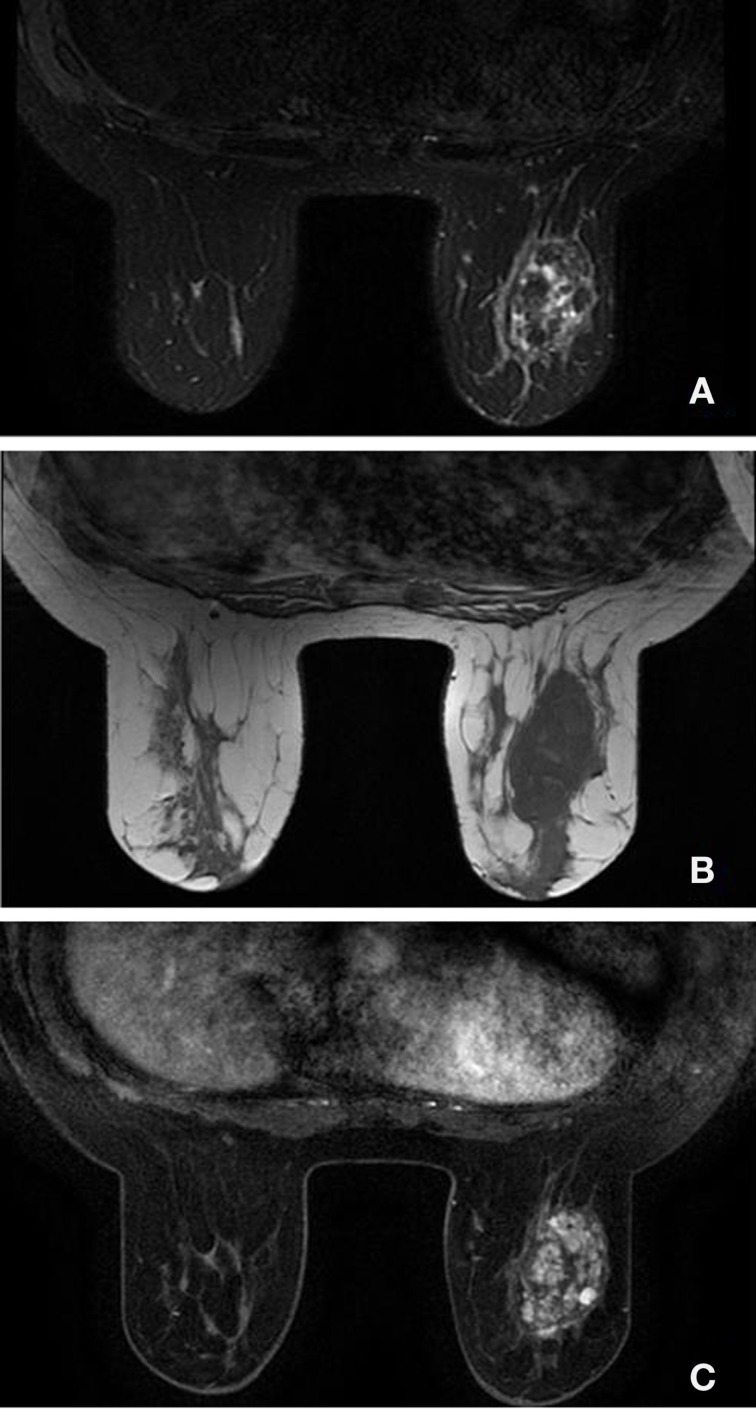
MRI showing the mass on the left breast with lobulated appearances. On T2-weighted MR image with fat saturation (A), the lesion demonstrates a heterogeneous appearance with areas of low and high signal. On T1-weighted MR image (B), the lesion shows a more homogeneous appearance with a hyposignal. On T1-weighted MR image with gadolinium and fat saturation (C), the mass demonstrates a heterogeneous enhancement, with a polylobulated appearance. No lymphadenopathies are seen.
